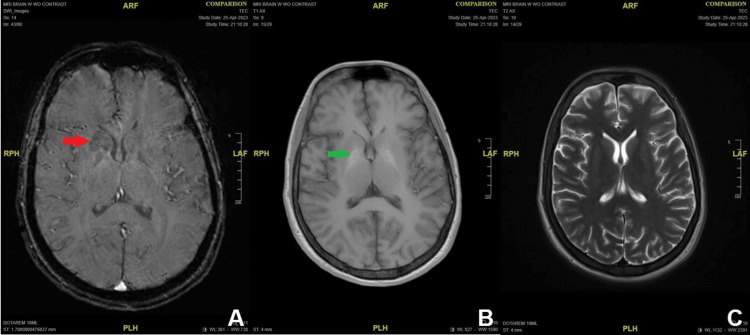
Magnetic resonance imaging (A) Mild restricted diffusion (red arrow) in the right caudate head extending into the anterior limb of internal capsule and the adjacent lentiform nucleus in susceptibility-weighted imaging (SWI). (B) Mild intrinsic globus pallidus hyperintensity (green arrow) in T1-weighted imaging. (C) Subtle associated asymmetric hyperintensity T2-weighted imaging
